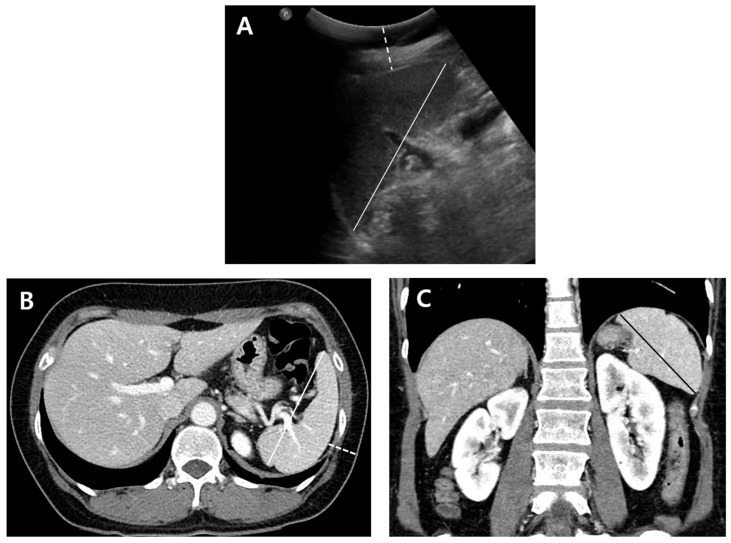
Measurement of spleen on US and CT in a 54-year-old woman. (A) Longitudinal diameter of the spleen in supine position (solid line) and the depth of the spleen from the skin (dotted line) are measured on US. (B) The longest diameter of the spleen on axial CT (solid line) and the distance of the spleen from the skin (dotted line) are measured. (C) The longest diameter of the spleen on coronal CT (solid black line) is measured. Splenic lengths measured on US (A), axial CT (B), and coronal CT (C) were 8.7 cm, 10.5 cm, and 8.9 cm, respectively. Depths of the spleen from the skin on US (A) and CT (B) were 2.1 cm and 2.3 cm, respectively.
